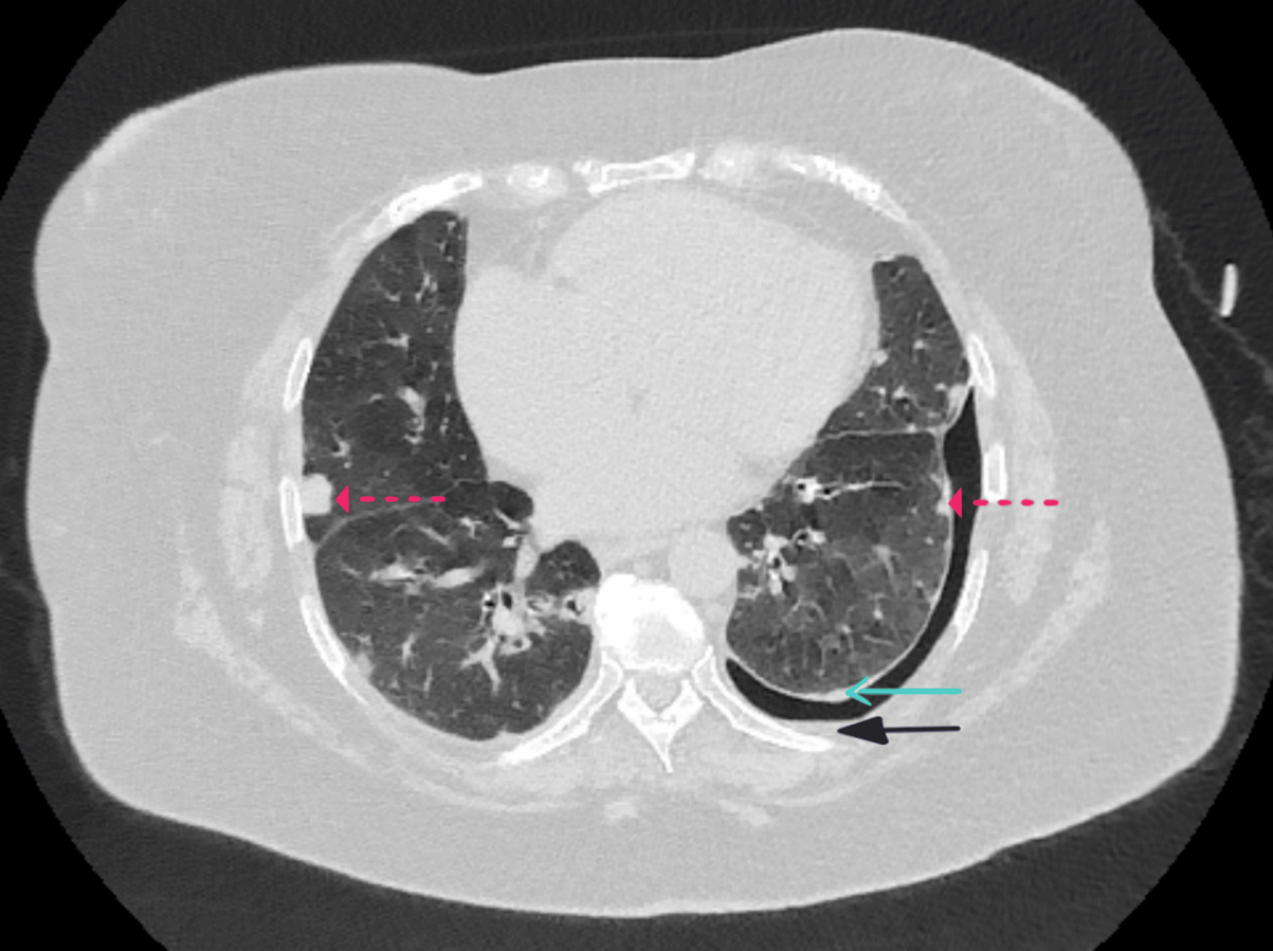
Axial view of repeat contrast-enhanced CT thorax demonstrating a 12 mm left-sided pneumothorax, minimal pleural effusion, pleural thickening, and stable subpleural rheumatoid nodules CT: Computed Tomography Solid blue arrow: Pleural thickening; Solid black arrow: Pleural effusion; Dotted red arrow: Rheumatoid nodule
